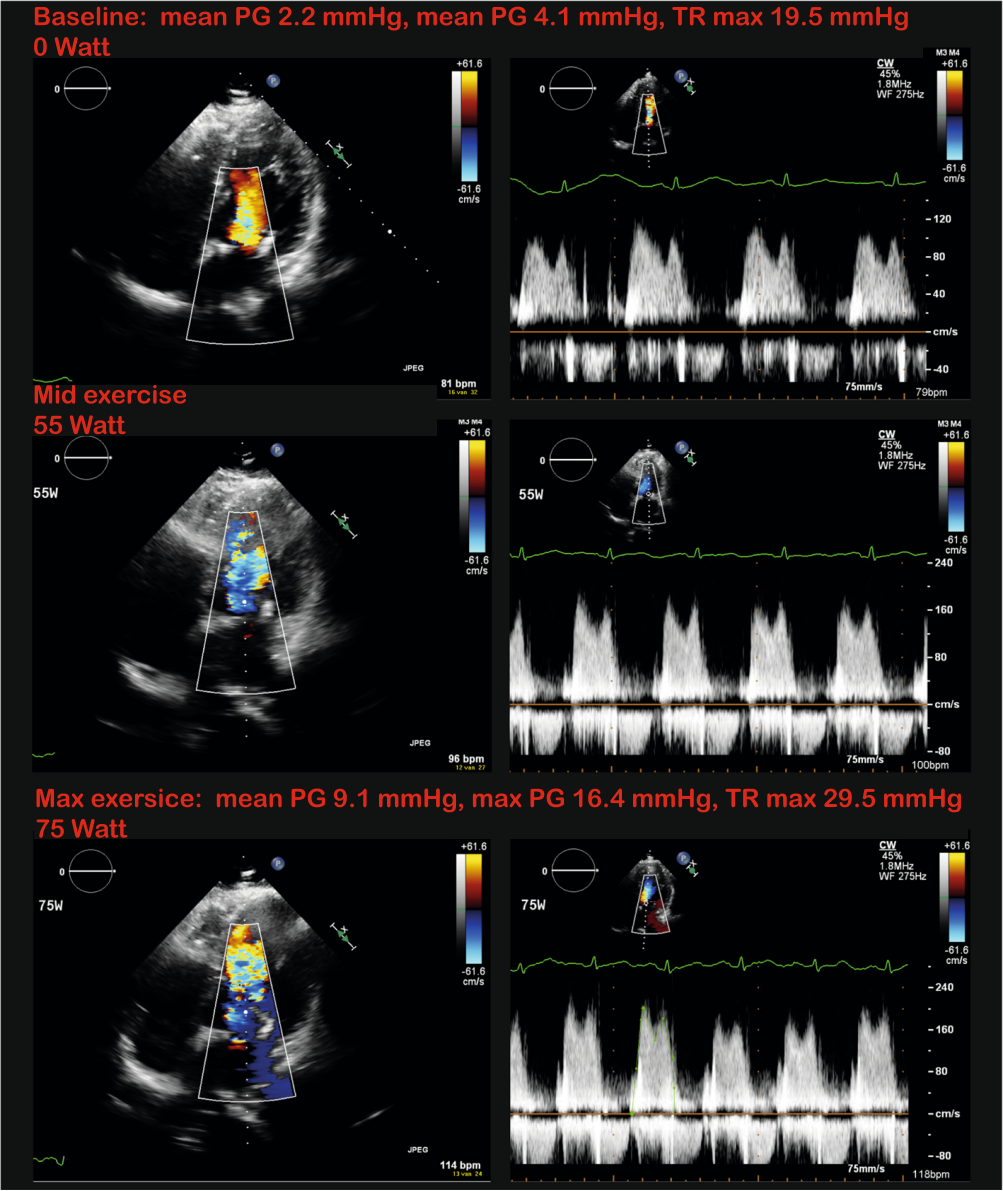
Stress echocardiography of mitral valve. SE performed with the Probefix in patient six. The patient had a history of mitral valve plasty due to P2 leaflet prolapse. Patient experienced progressive dyspnoea, fatigue and weight gain after surgery. SE was performed to assess the mitral valve pressure gradient during exercise, which was not elevated. At baseline and at peak exercise, right ventricular filling pressures were also assessed with continuous wave Doppler of the tricuspid valve
